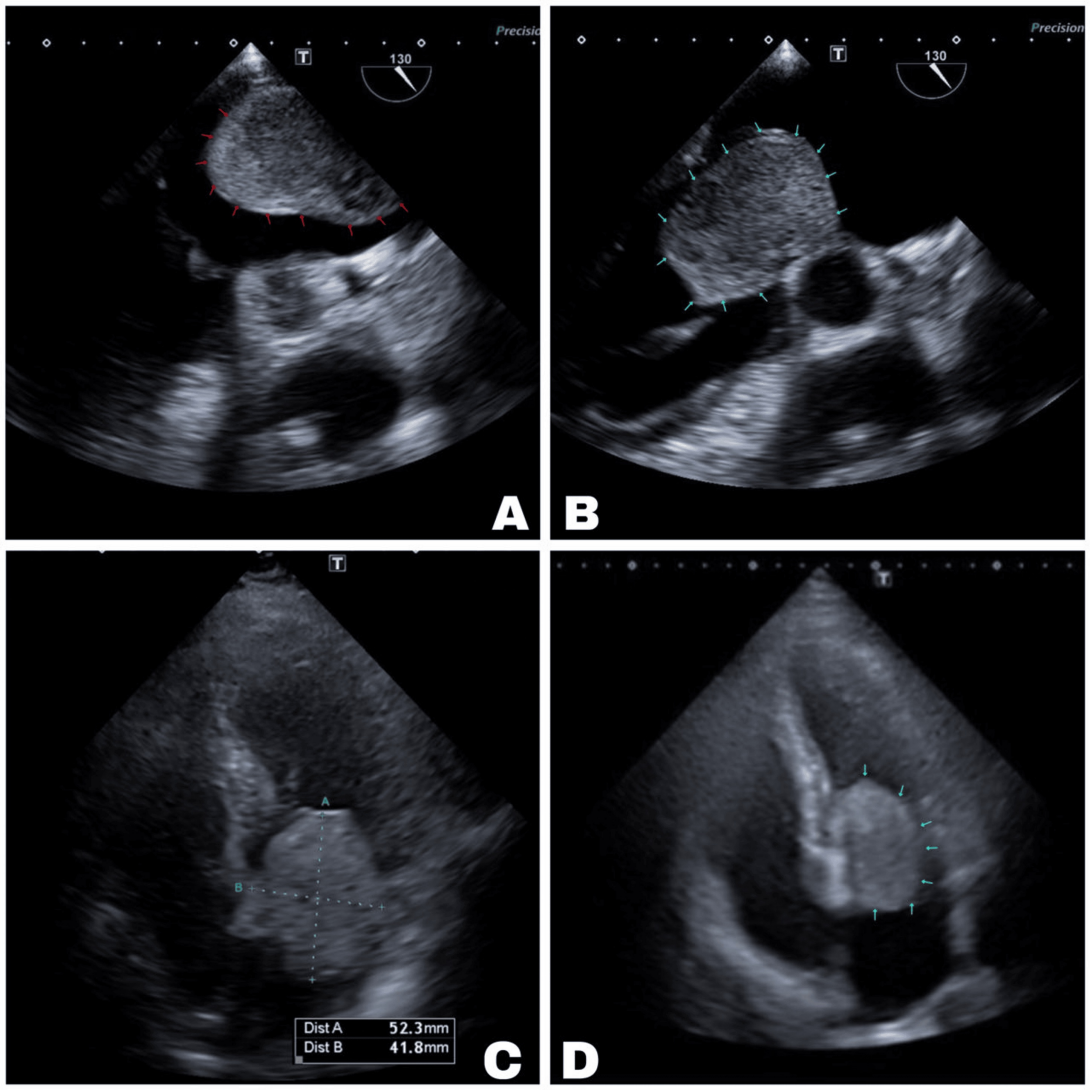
Echocardiographic Findings of an Intra-Atrial Mass A. Transesophageal echocardiogram, a parasternal long-axis view at 140 degrees, demonstrates an echogenic mass occupying the left atrium, with its outline highlighted by red arrows. B. Transesophageal echocardiogram, a parasternal long-axis view at 130 degrees, reveals an echogenic mass prolapsing through the mitral valve into the left ventricle, with its silhouette delineated by light blue arrows. C. Transthoracic echocardiogram, apical four-chamber view at the mitral valve level, shows an echogenic mass measuring 52.3 mm x 41.8 mm. D. Transthoracic echocardiogram, apical four-chamber view, depicts an echogenic mass prolapsing through the mitral valve into the left ventricle, with its outline marked by light blue arrows.
